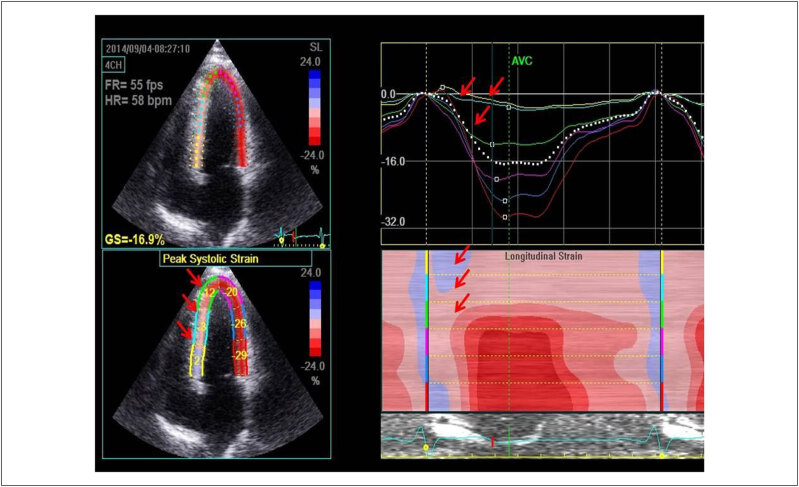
Representação da avaliação do strain longitudinal em paciente com CMH. Observa-se redução dos valores do strain longitudinal nos segmentos (basal, médio e apical) hipertróficos da parede septal (setas) no corte apical 4 câmaras. Fonte: Arquivo pessoal dos autores.
